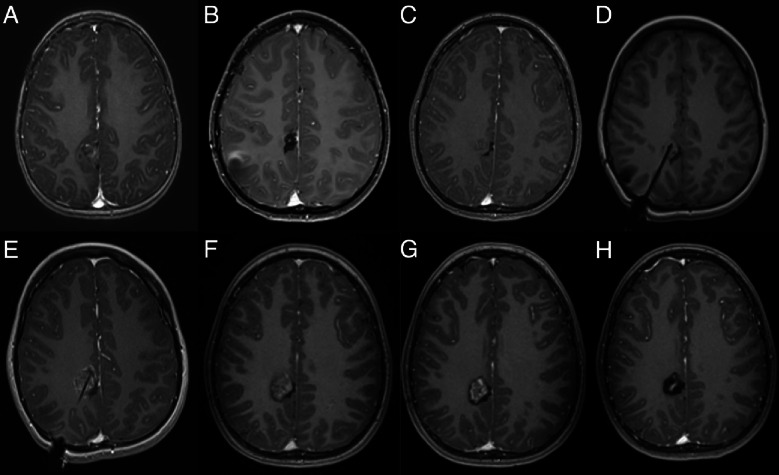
Serial axial T1-enhanced MR images of patient #1. A At diagnosis, B after resection, C tumor recurrence, D intraoperative image showing the approach to the tumor, E complete ablation of the tumor at the end of surgery, F, G continues contrast enhancememt 3 and 6 months after surgery, H 2.5 years after surgery, demonstrating good local control
